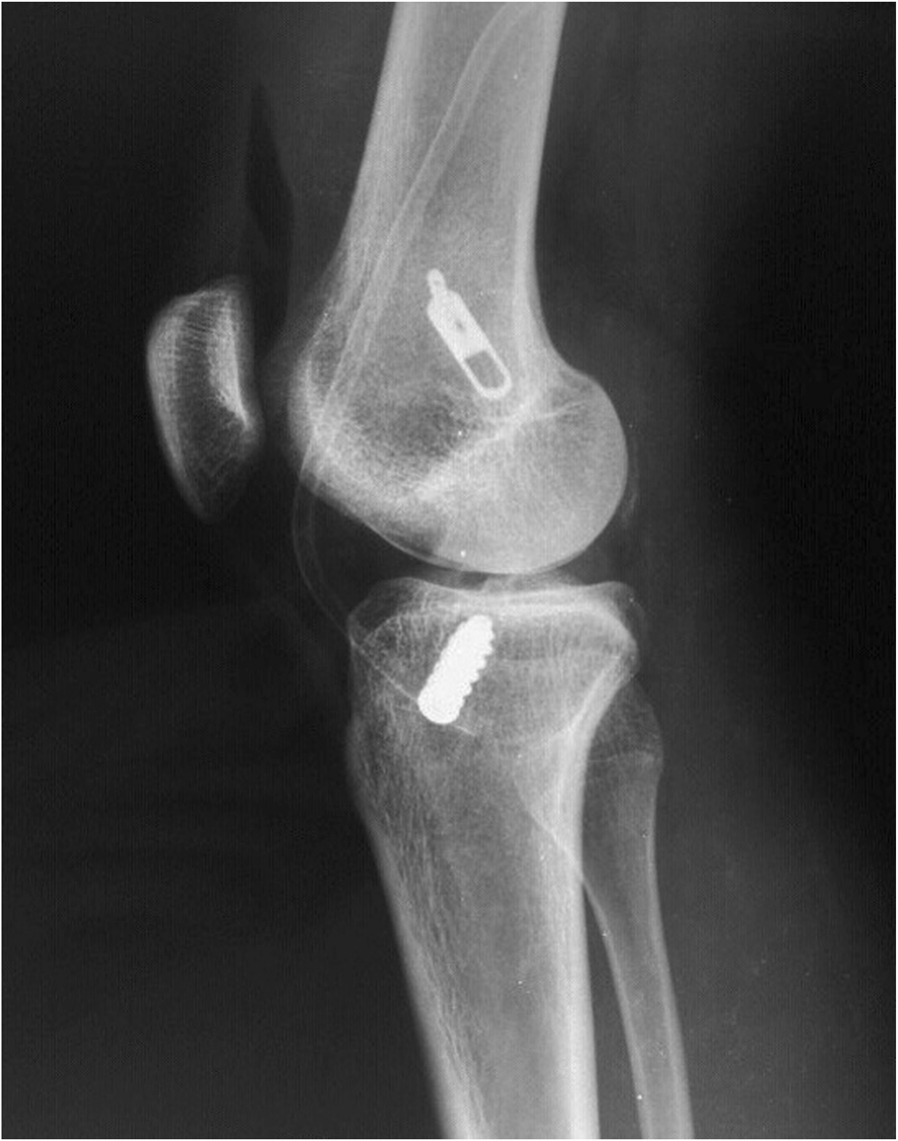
Postoperative profile radiograph of an anterior cruciate ligament reconstruction (same case as that shown in Figure
